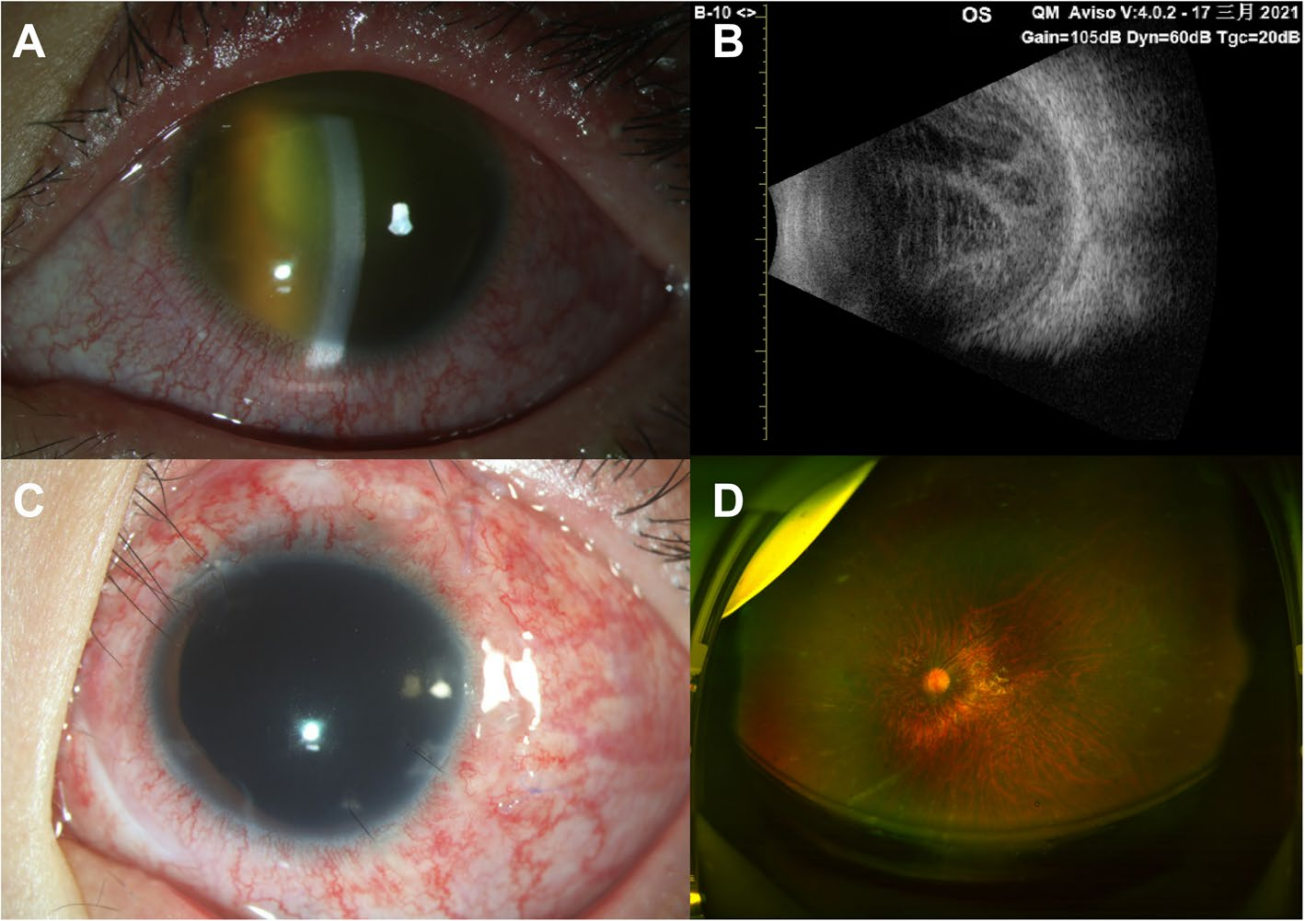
For patient #1. A anterior segment photography before vitrectomy. B B-ultrasound before vitrectomy. C anterior segment photography at the latest follow-up. D Ultra-wide angle fundus photography at the latest follow-up
